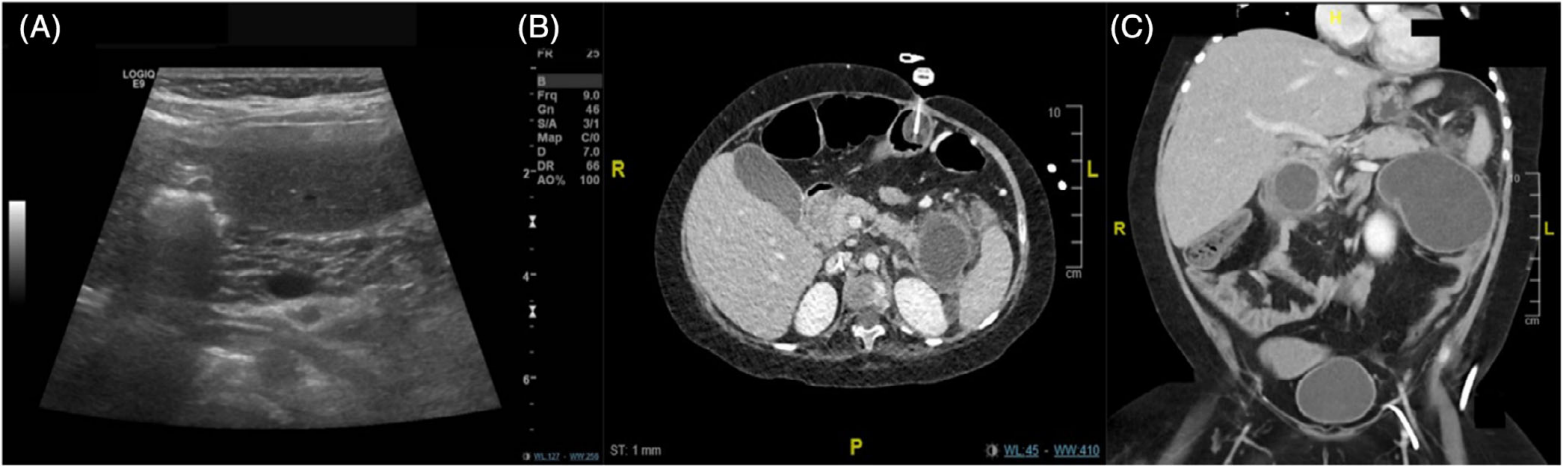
Pancreatic imaging findings. A, Case 2: abdominal ultrasonography: The visualized pancreas is diffusely heterogenous in echotexture with multiple foci of parenchymal low echogenicity in keeping with chronic pancreatitis. B,C Case 3: Contrast enhanced CT abdomen: evidence of an inflammatory thick‐walled pseudocyst in the region of the uncinate process of the pancreas (34 × 34 mm). In addition, there is dilatation and ectasia of the pancreatic duct and a large pseudocyst demonstrated in the pancreatic tail. This extends out to Gerota's fascia on the left side and has a more linear course adjacent to the descending colon to terminate in a very large pseudocyst (measuring 71 × 75 × 87 mm) which extends toward the anterior abdominal wall and reaches almost into the left side of the pelvis. Small inflammatory pseudocysts in the region of the splenic hilum and greater curve of the stomach are seen
